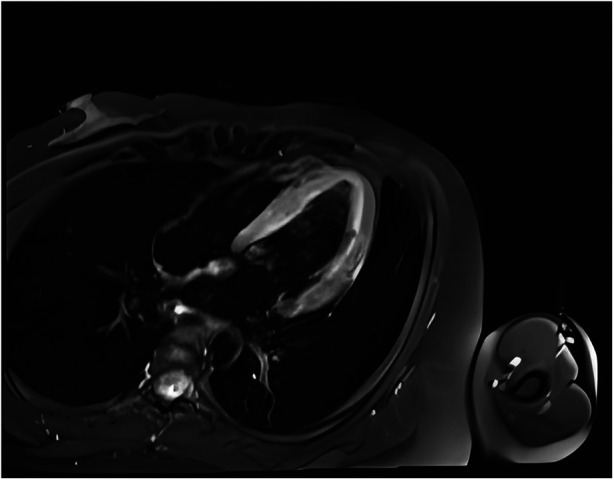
Cardiac magnetic resonance T2-weighted imaging shows diffuse hypersignal in the left ventricular wall.
